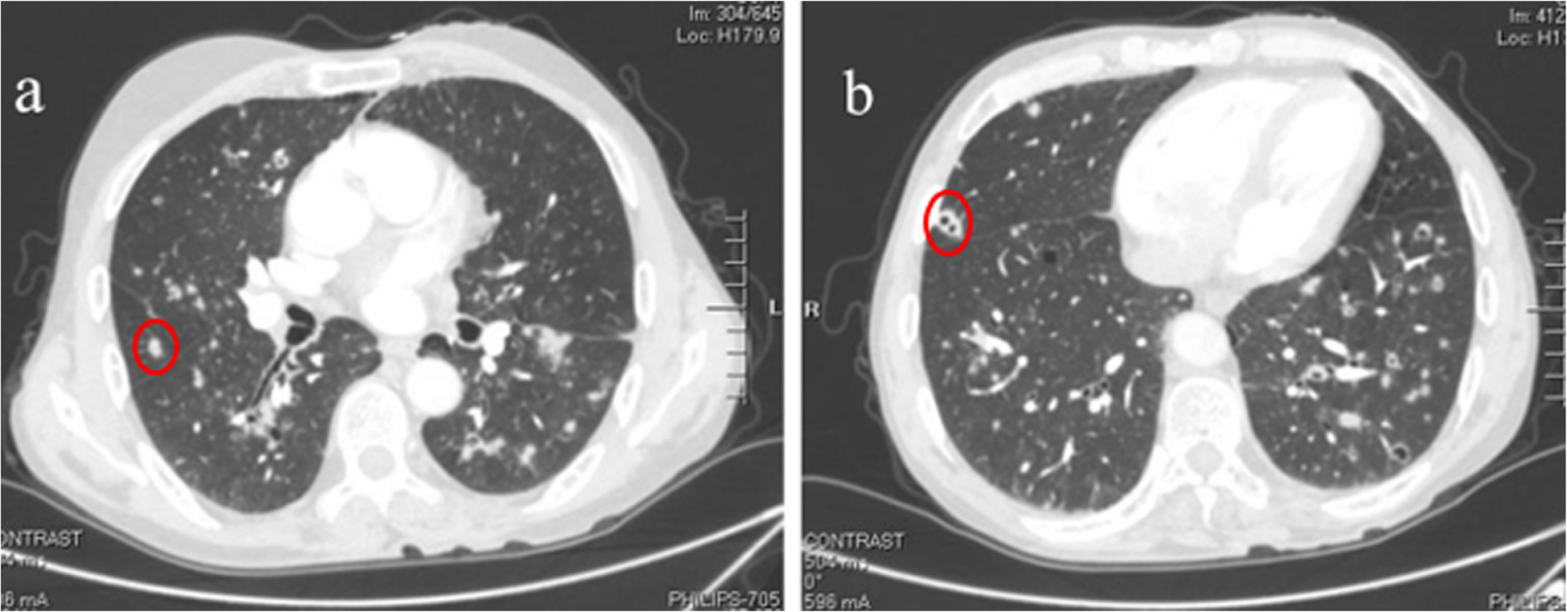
Chest enhanced CT:multiple nodules in both lungs, some of which had cavities. a The first lesion we resected. b The second lesion we resected
